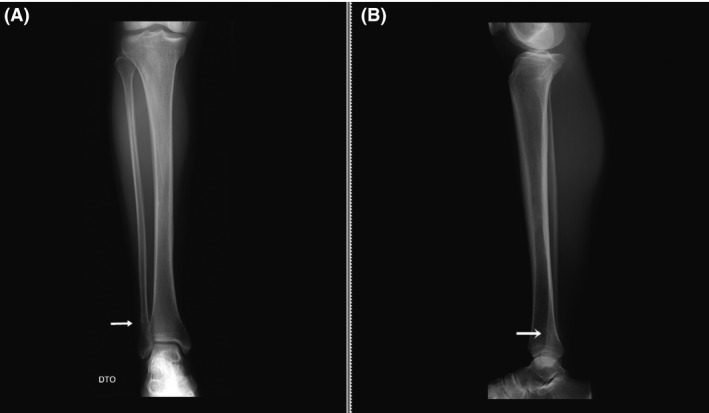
Right leg radiography, AP (A) and lateral (B) incidences, showing fibular fracture, with hypertrophic callus (arrow).
